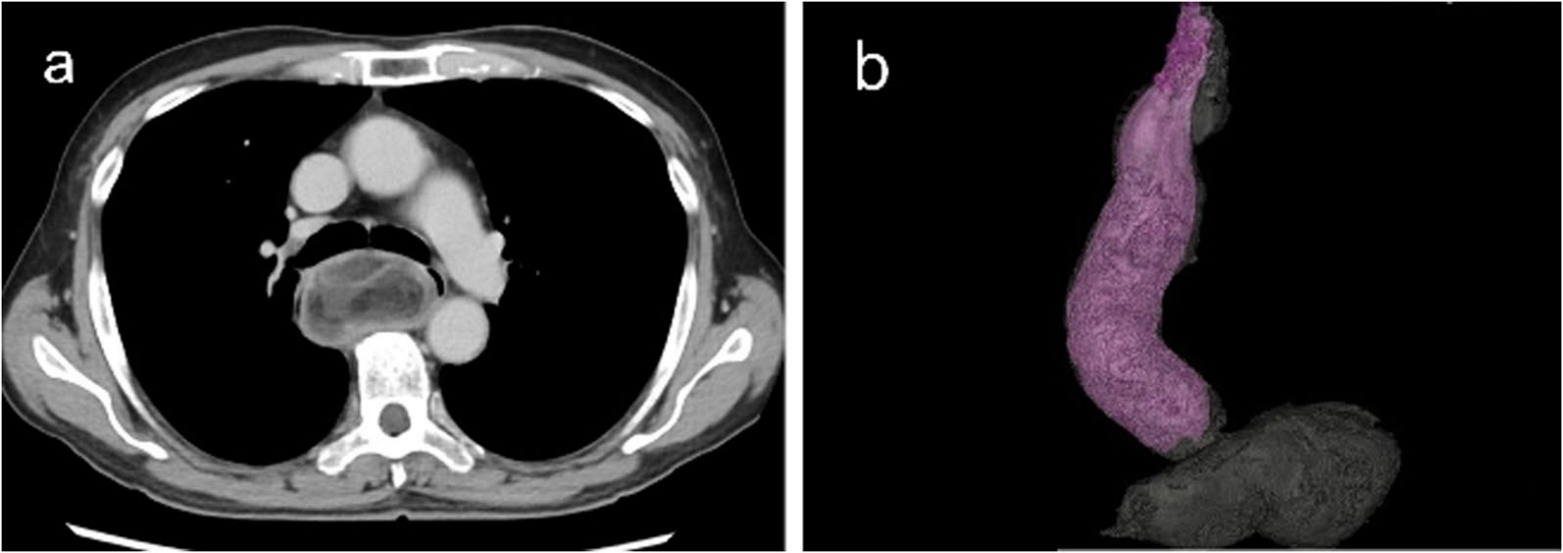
a Preoperative contrast-enhanced CT showed a large pedunculated tumor hanging in the cervical esophagus. The inside of the tumor was heterogeneously contrasted, with a mixture of fatty, fibrous and vascular components. b 3D-CT showed that the tumor was an intraluminal polypoid tumor
